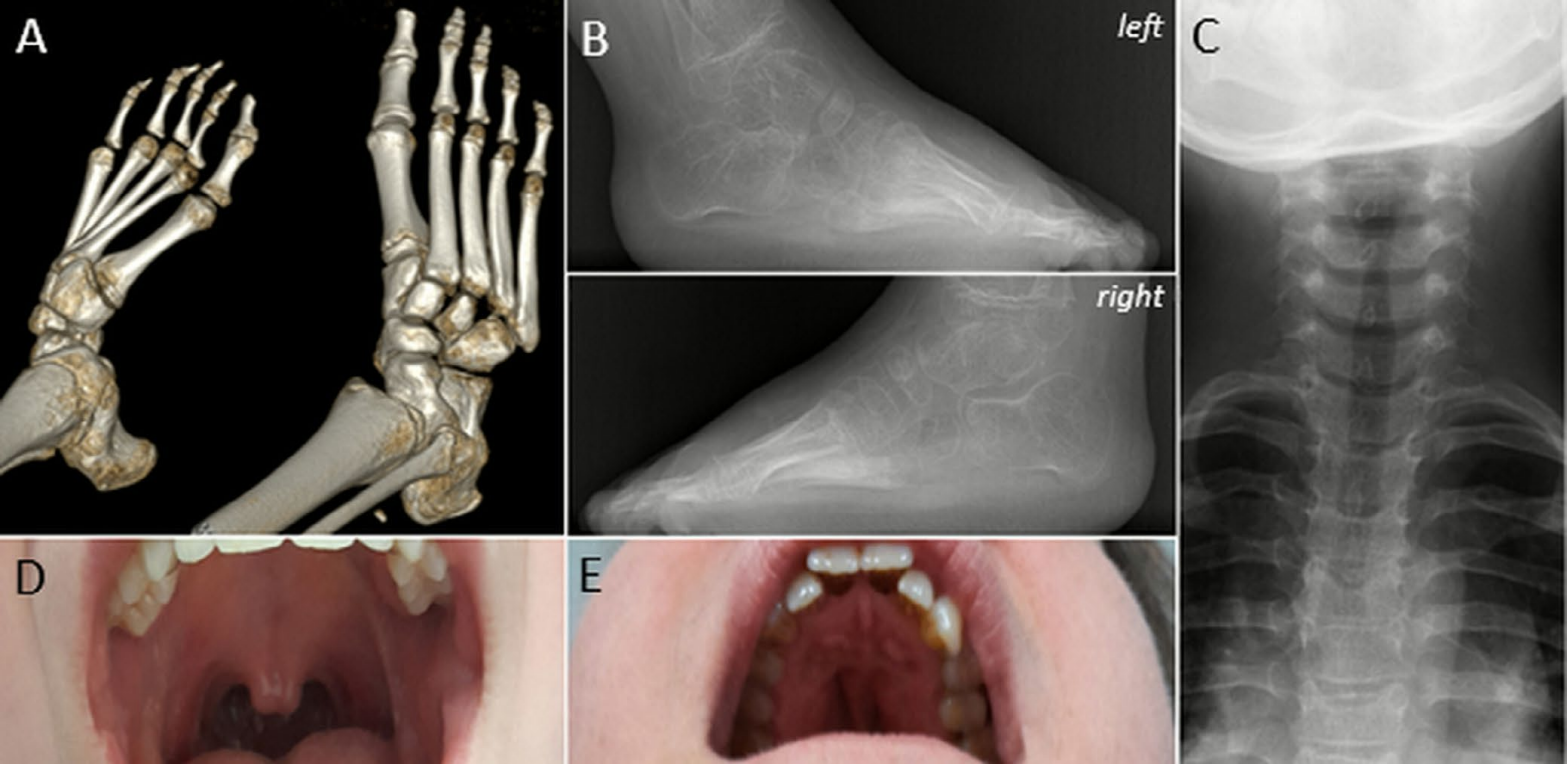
(A) Foot CT scan reconstruction (proband at age 11); (B, C) Foot and cervicothoracic spine radiographs (brother at age 6) showing absence of skeletal fusion. (D) Bifid uvula and a discrete posterior submucosal cleft palate in the sister. (E) Sequelae of cleft palate surgery in the mother. [Colour figure can be viewed at wileyonlinelibrary.com]
